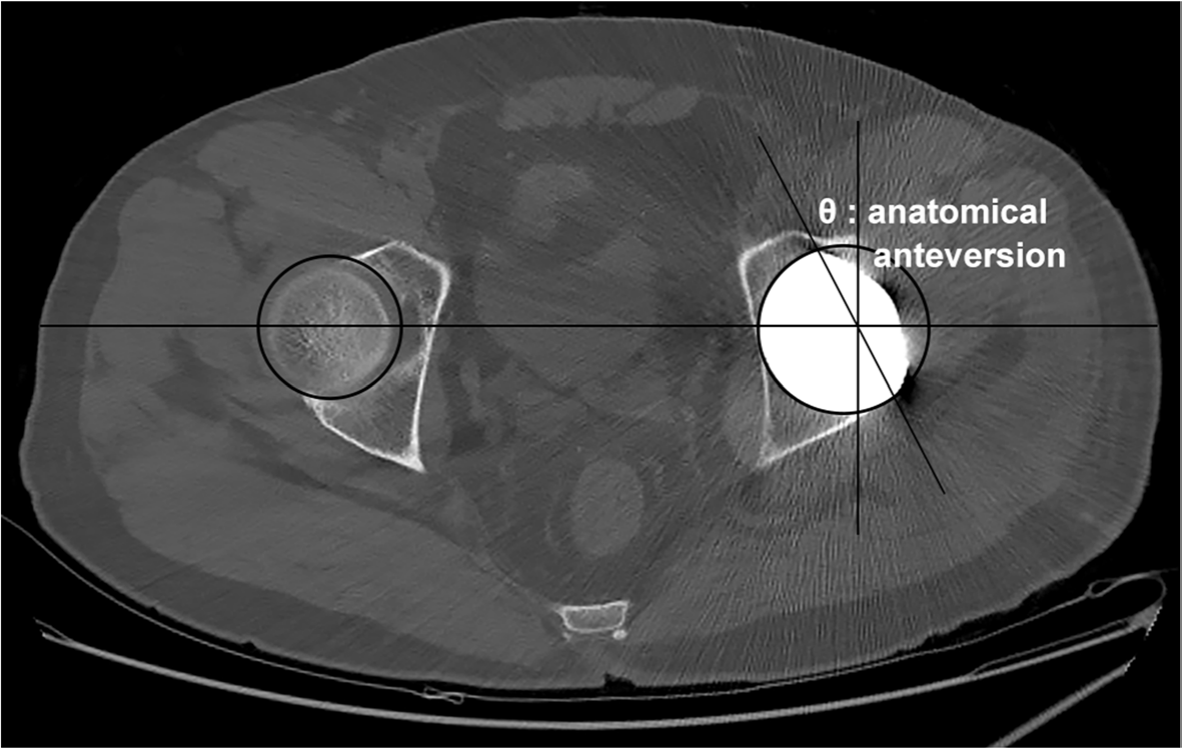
Assessment parameters for measuring anatomical anteversion on CT scans by the method from Murray’s concept
